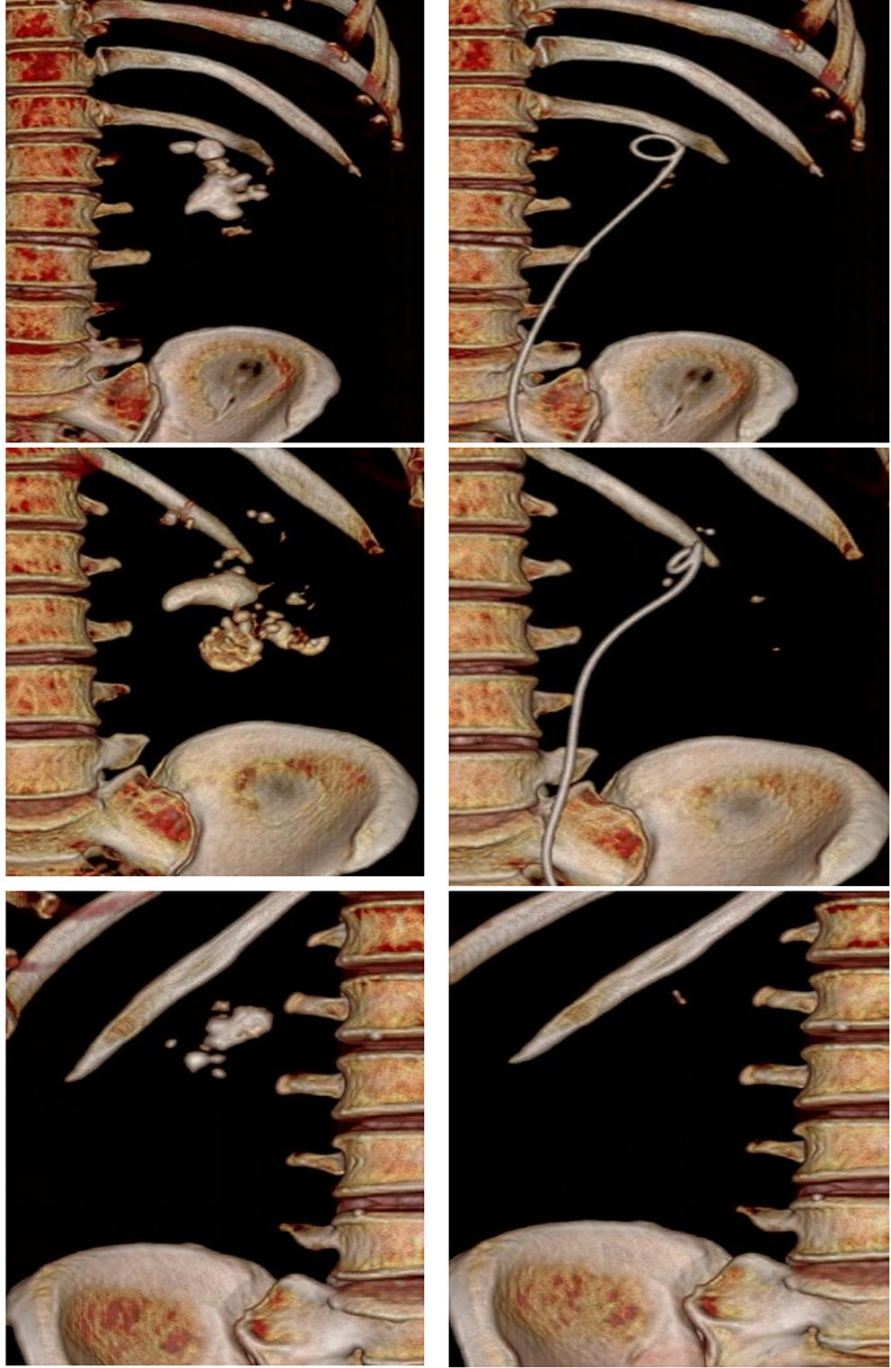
CT scan (3D) before and after treatment with flexible ureteroscopy and Thulium fiber laser lithotripsy with residual fragment (RF)
